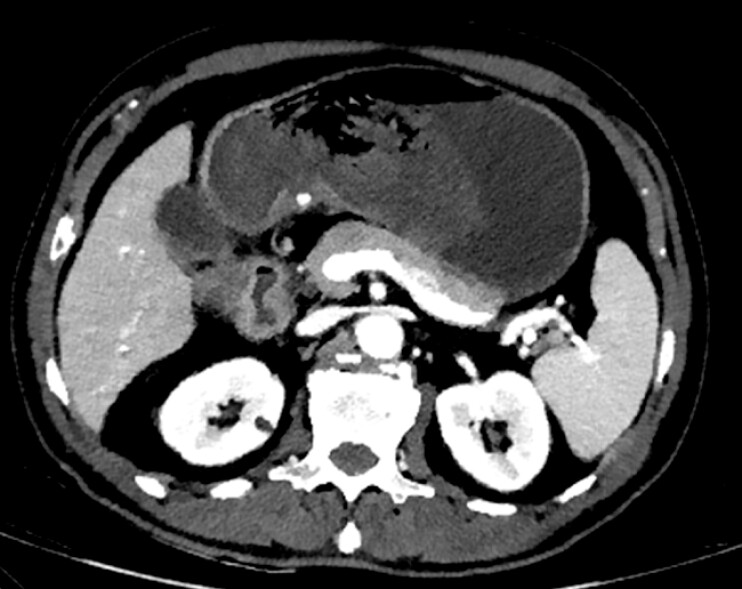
CT image of the hyperdense spherical lesion located in the lesser curvature.
